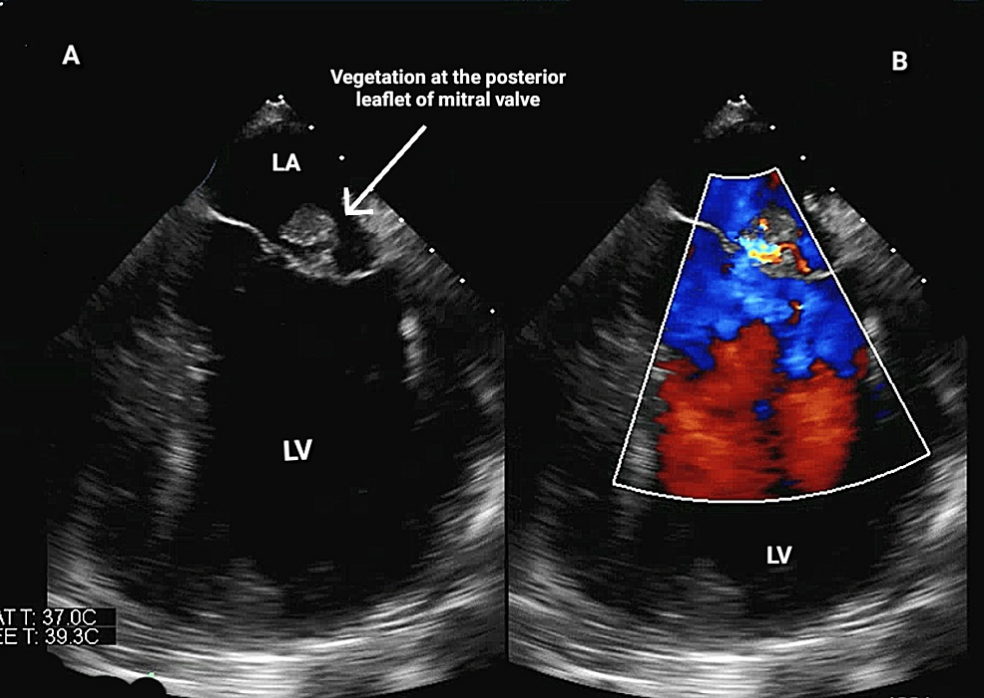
(A) TEE showing vegetation attached to the posterior leaflet of the mitral valve. (B) TEE showing mitral regurgitation with suspicion of mitral valve perforation LA, left atrium, LV, left ventricle; TEE, transesophageal echocardiography
